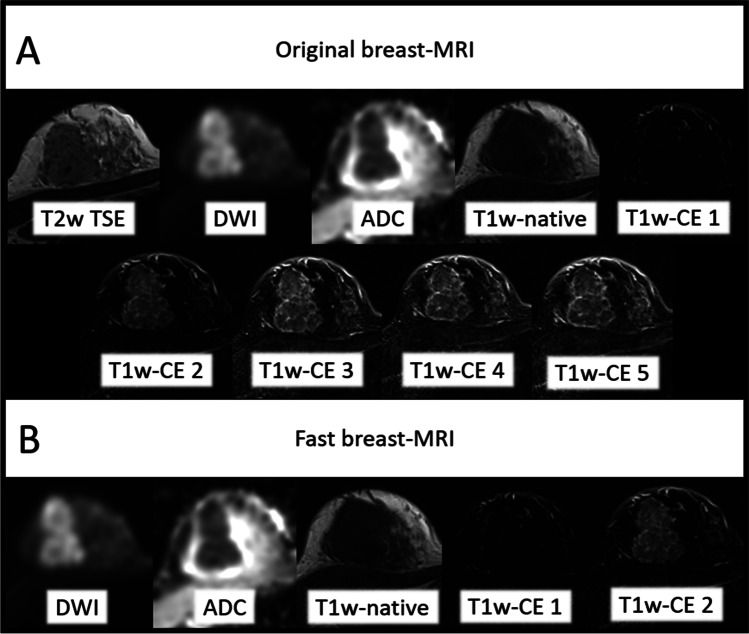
MRI dataset of a 39-year-old breast cancer patient with a T2_ts_ breast cancer lesion in the right breast. Good diagnostic detectability in (A) the original comprehensive breast MRI protocol and in (B) the fast breast MRI protocol without losing essential information
